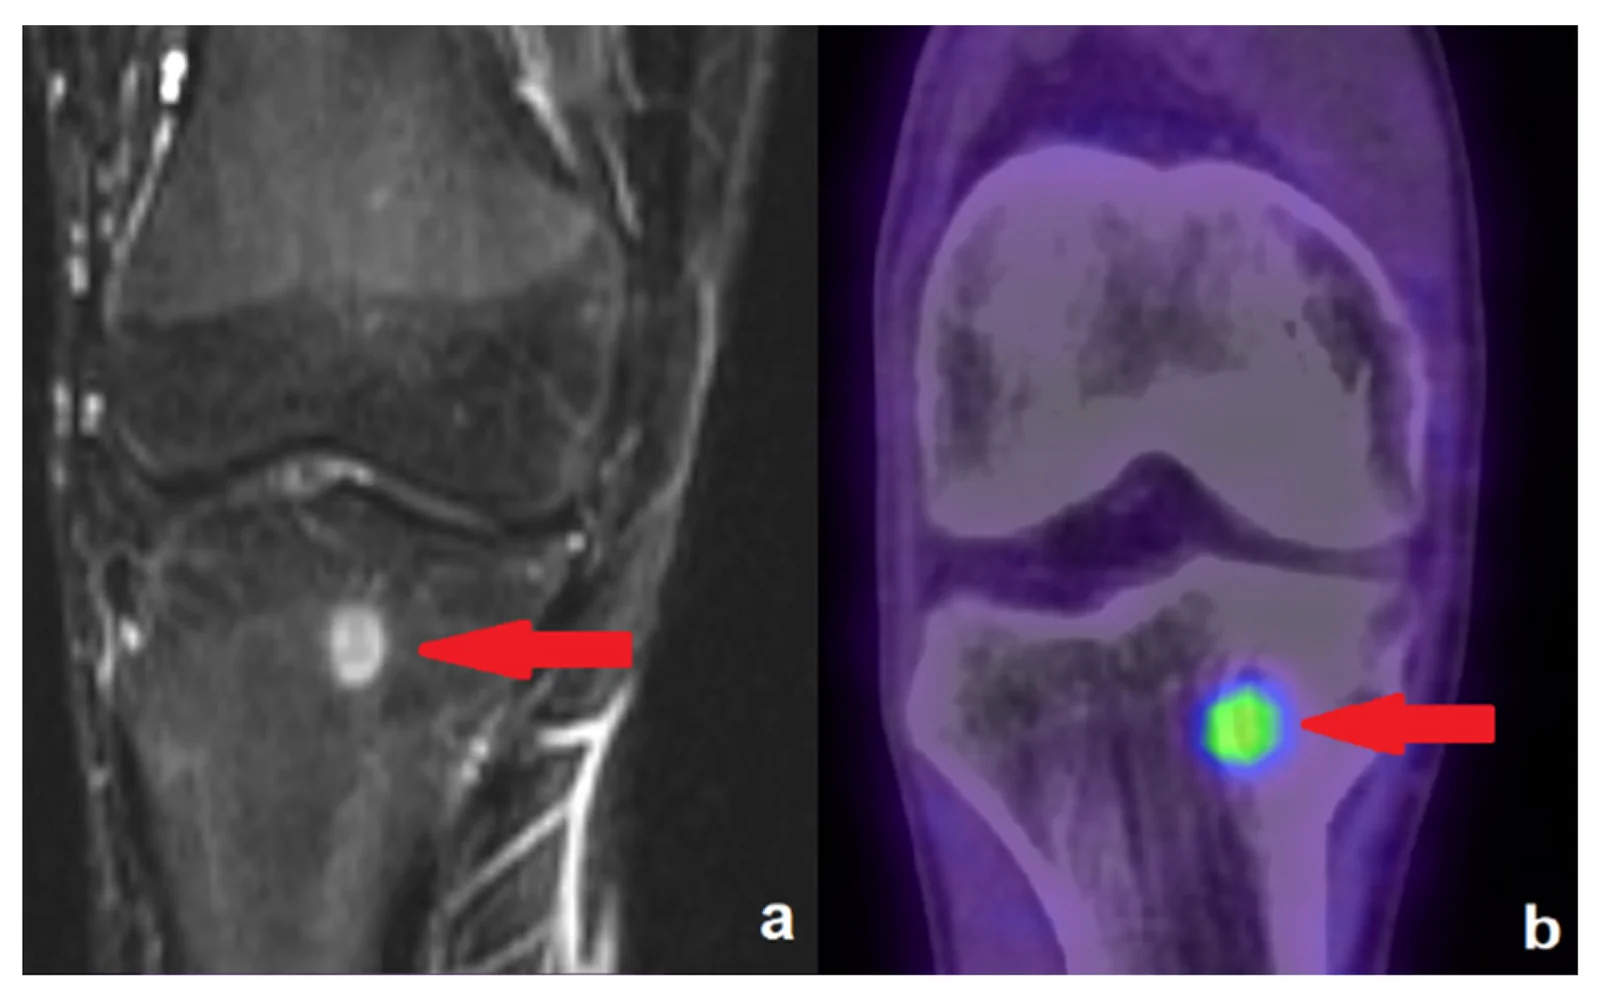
MR image (coronal T2-weighted TIRM sequence) demonstrating a focal hyperintense lesion in the proximal right tibia (red arrow, (a)) with an increased FDG uptake on the corresponding PET/CT image (color-coded as in Figure 2, (b)).
